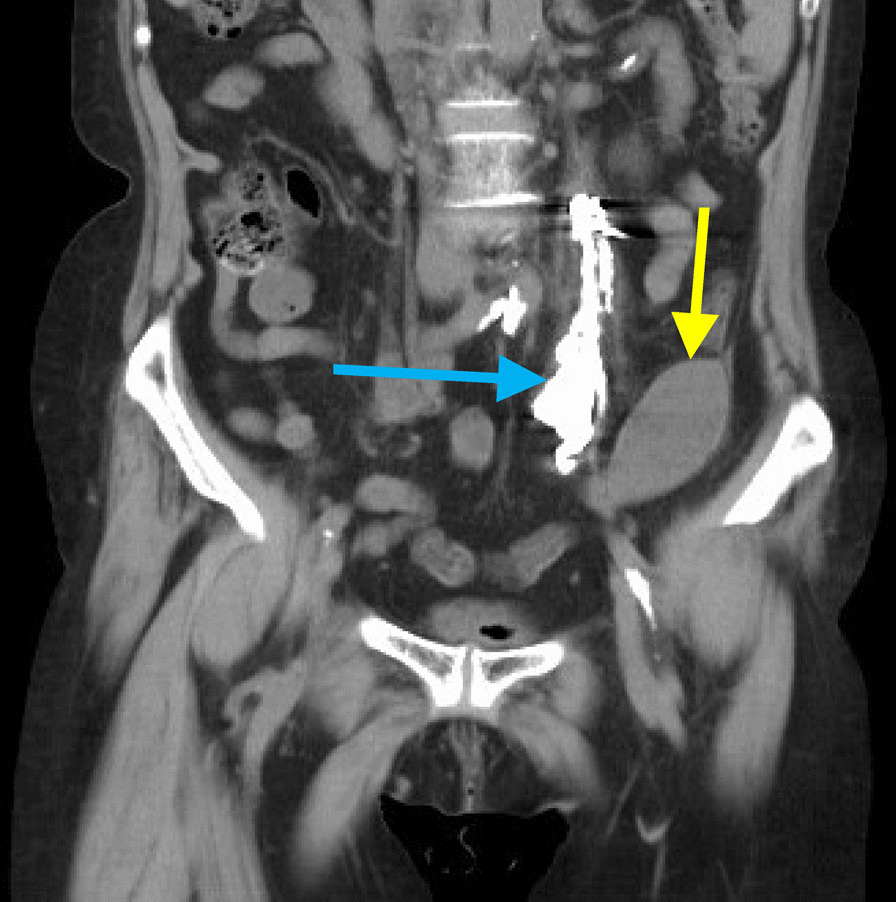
Computed tomographic image on the second day after embolization. The yellow arrow indicates the hematoma, which shrank, and the blue arrow indicates the embolized left ovarian vein
